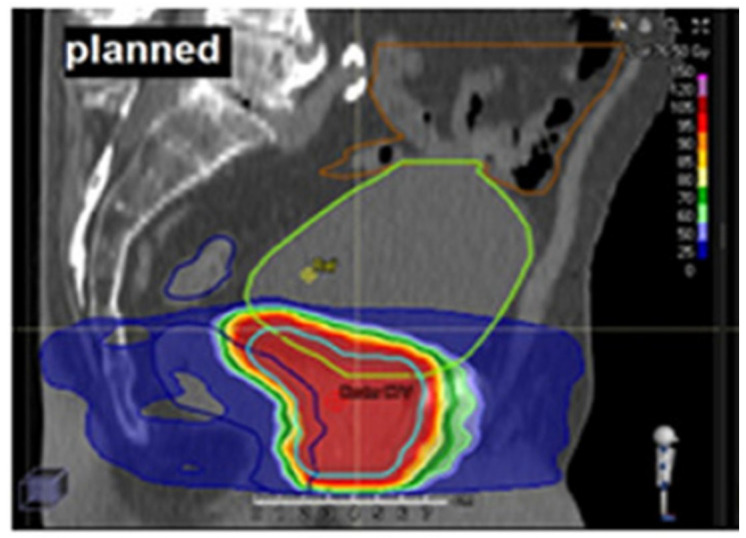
Example of a sagittal computed tomography (CT) image showing the planned radiation dose distribution to the clinical target volume (CTV), bladder, and rectum. For patients with low-risk prostate cancer, the CTV included only the prostate, whereas in intermediate-risk cases, it also encompassed 10–15 mm of the proximal seminal vesicles. A 7 mm margin was added to the CTV to define the planning target volume (PTV). The prescribed dose was 76.50 Gy, delivered in 34 fractions of 2.25 Gy each. The bladder and rectum, considered organs at risk (OARs), are contoured in light green and dark blue, respectively. Adapted from Bostel et al. [4], an open access article distributed under the Creative Commons Attribution License (CC BY).
